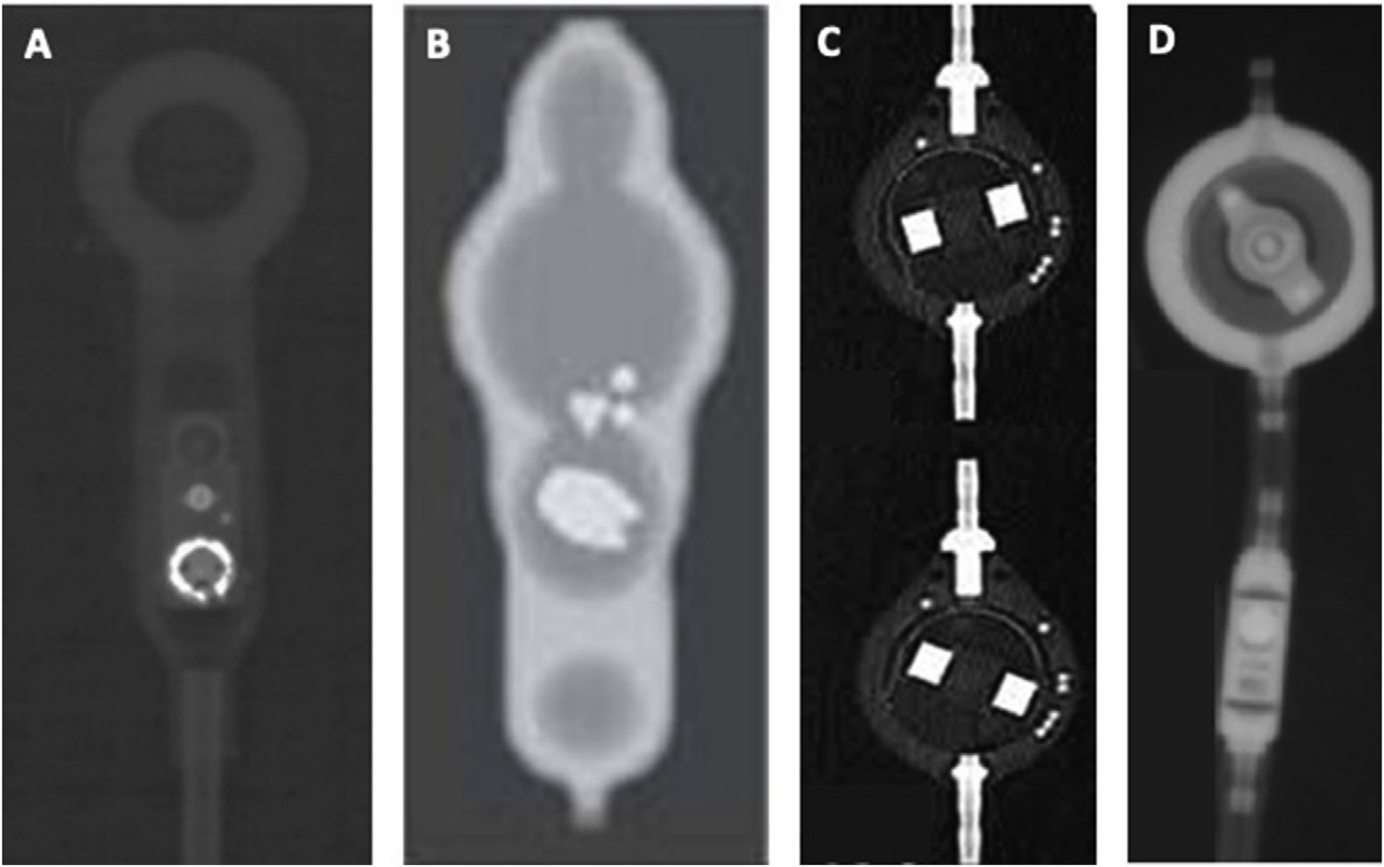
Radiographic appearances of some commonly used shunt valve systems, Codman Hakim (A), Medtronic Strata (B), Sophysa Sophy SM6 (C), Aesculap Miethke ProGAV (D). Figures adapted from Lollis et al., 2010 and Handbook of Neurosurgery, Greenberg, 2010 [3,4].
